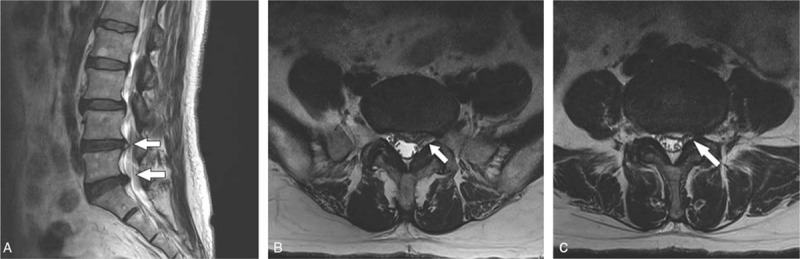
Sagittal T2-weighted magnetic resonance imaging (MRI) showing L4–5 and L5–S1 disc herniation (A). Axial view of MRI shows left foraminal disc protrusion at L4–5 (B), left subarticular disc protrusion at L5–S1 (C).
